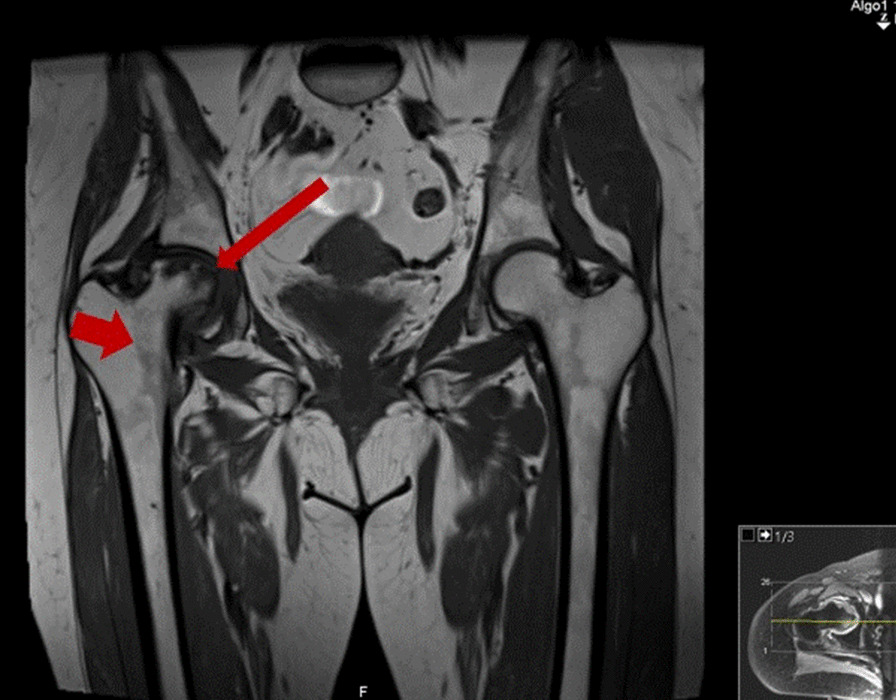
Magnetic resonance imaging (MRI) of the hip. Small to moderate articular effusion in the right hip joint, with synovitis and marked dysmorphia of the femoral head with flattening of its superior slope (*thin red arrow*), and peripheral, subcortical edema of the femoral head involving almost all of the articular surface, delimited by a well-defined serpiginous line (*thick red arrow*). In the context of the given patient, these findings should represent avascular necrosis of the femoral head, probably post-traumatic, in stage IV
